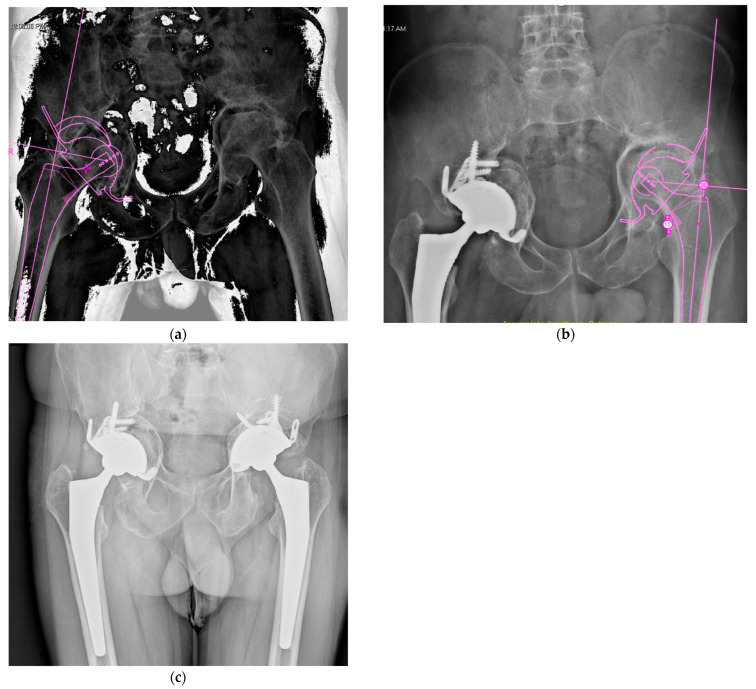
Preoperative planning images for right (a) and left (b) total hip arthroplasty showing the extent of acetabular bone loss and implant positioning strategy. (c) Postoperative radiograph at the 24-month follow-up.
